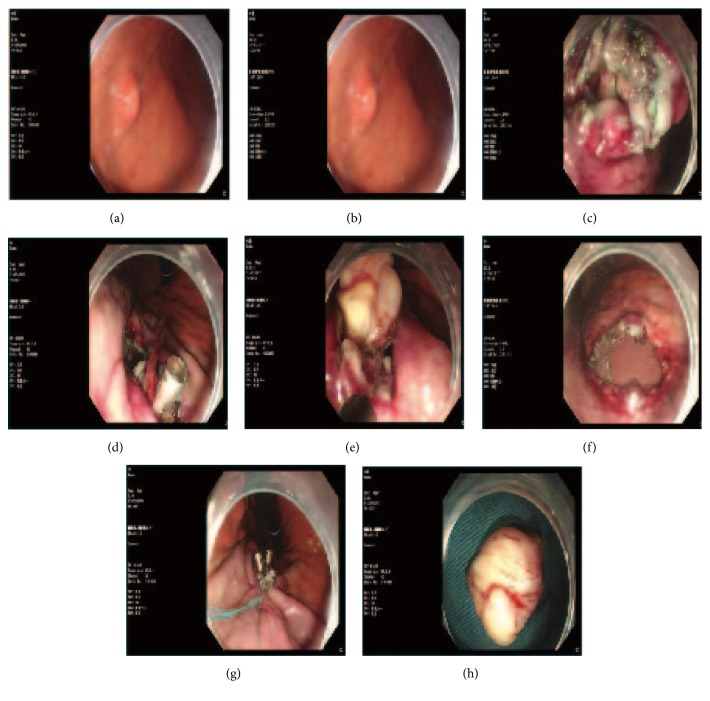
ESD with dental floss clip traction. (a) A bump was seen in the anterior gastric corpus wall (endoscopic ultrasound showed it originated from the muscular layer and grows extraluminally, 2.0cm in diameter), labeled with Hook Knife. (b) The mass was showed after Hook knife precutting the mucous layer. (c) Strip off the mass. (d) The mass was pulled by the dental floss clip. (e) The lesion clearly exposed with the dental floss traction. The lesion was easier to remove en bloc with hook knife. (f) The post-ESD wound has no defect left. A large perforation was seen. (g) The wound was large and closed with nylon loop pouch-suture through a single channel endoscope. (h) The tumor.
